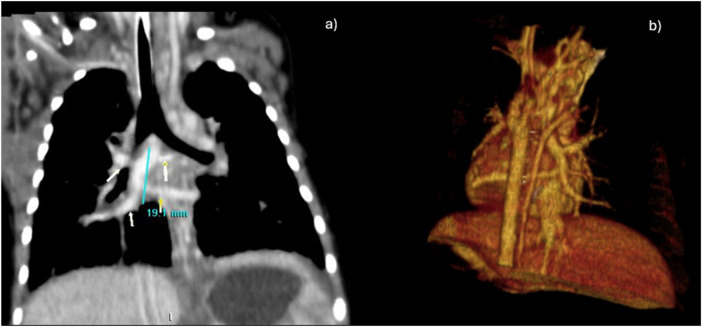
Panel (a) shows a grayscale coronal CT scan of the thorax with white and yellow arrows indicating anatomical landmarks of the pulmonary veins, and a blue measurement line labelled almost 20 mm (blue line) distance between the upper and lower veins. Panel (b) displays a 3D volume-rendered colour reconstruction of the same region, highlighting the thin vertical vein (diameter 4 mm), located right paravertebrally, into which the pulmonary veins drained.
